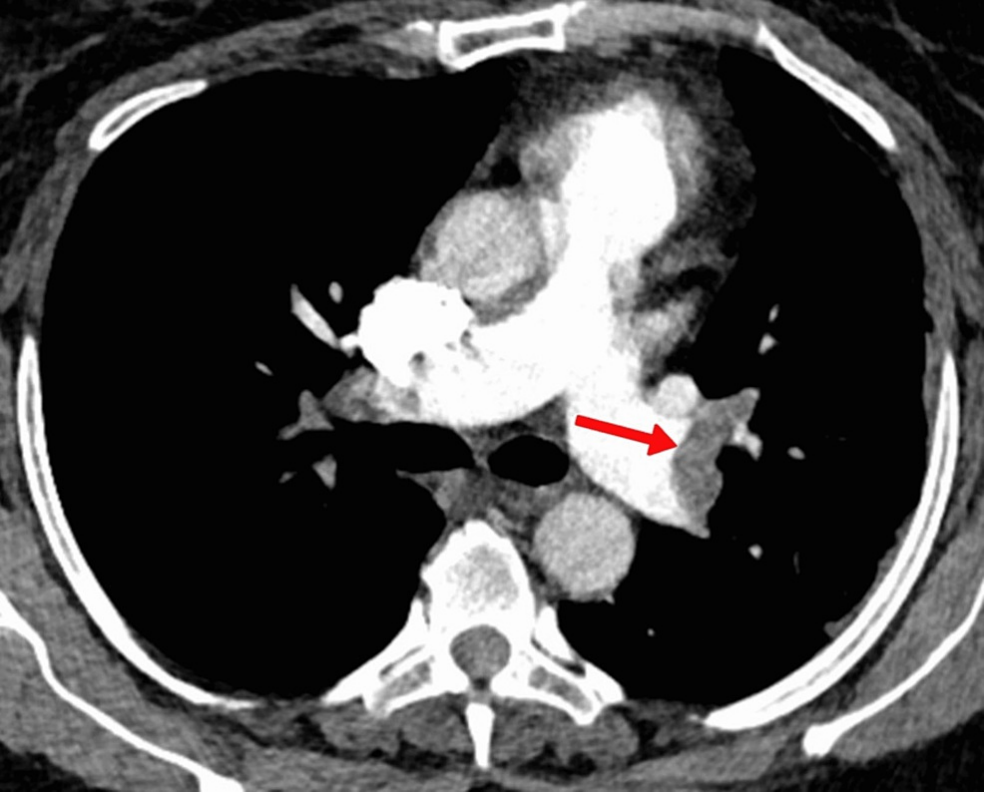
CT angiogram of the chest showing left main pulmonary artery thrombus
